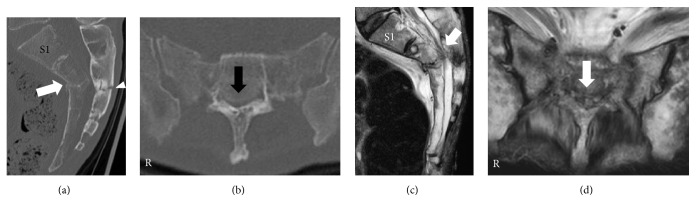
Reconstructed computed tomography (CT) and magnetic resonance images of sacrum at first hospital presentation*. (a) Sagittal reconstructed CT image. (b) Axial CT image at S2-3 level. (c) Sagittal plane on a T2-weighted image. (d) Axial plane on a T2-weighted image. Fractures in the sacral vertebral body (white arrow) and lamina (white arrowhead) can be observed (a). Radiological findings were consistent with a Roy-Camille classification type I fracture [5]. Sacral canal was narrowed by the protruding sacral vertebral body (black arrow) (b). This radiological finding was diagnosed as a Denis Zone III sacral fracture [15]. Sagittal and axial plane on T2-weighted images showed that sacral nerves were compressed by the vertebral body (white arrow) (c, d).
